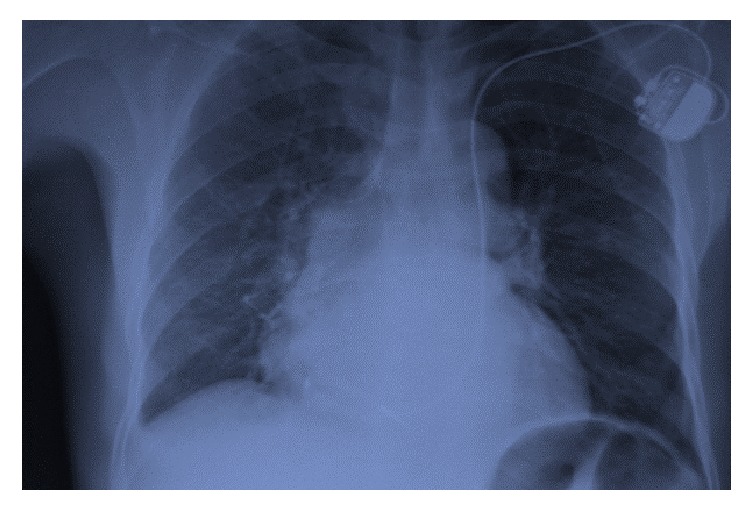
Chest X-ray seen from the front showing the cardiac stimulation lead descending into the LSVC, making a loop in the right atrium before being fixed in the right ventricle, just behind tricuspid valve.
